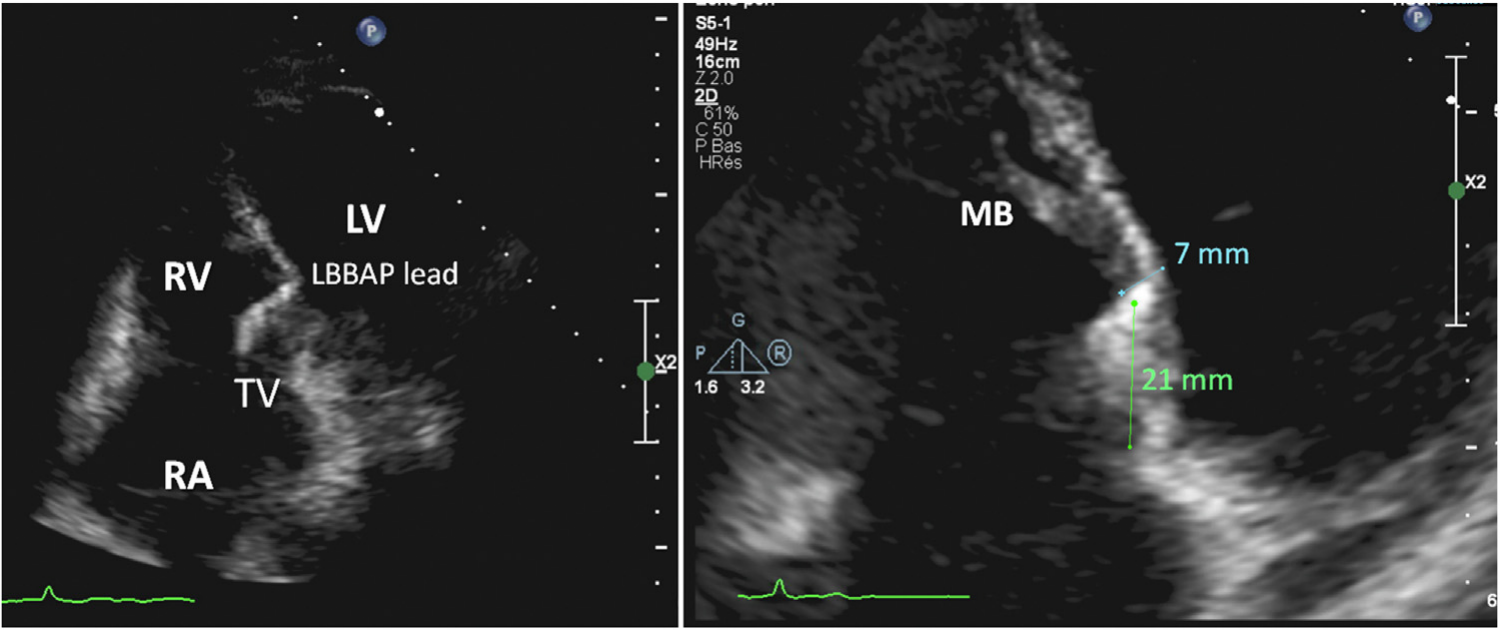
Left panel: Echocardiogram in the apical 4-chamber view showing left bundle branch area pacing (LBBAP) lead position. Right panel: Zoom showing lead tip in the left ventricular septal subendocardium, with insertion near the base of the moderator band (MB), 21 mm from the septal leaflet of the tricuspid valve (TV). Thickness of the interventricular septum was only 7 mm. LV = left ventricle; RA = right atrium; RV = right ventricle.
